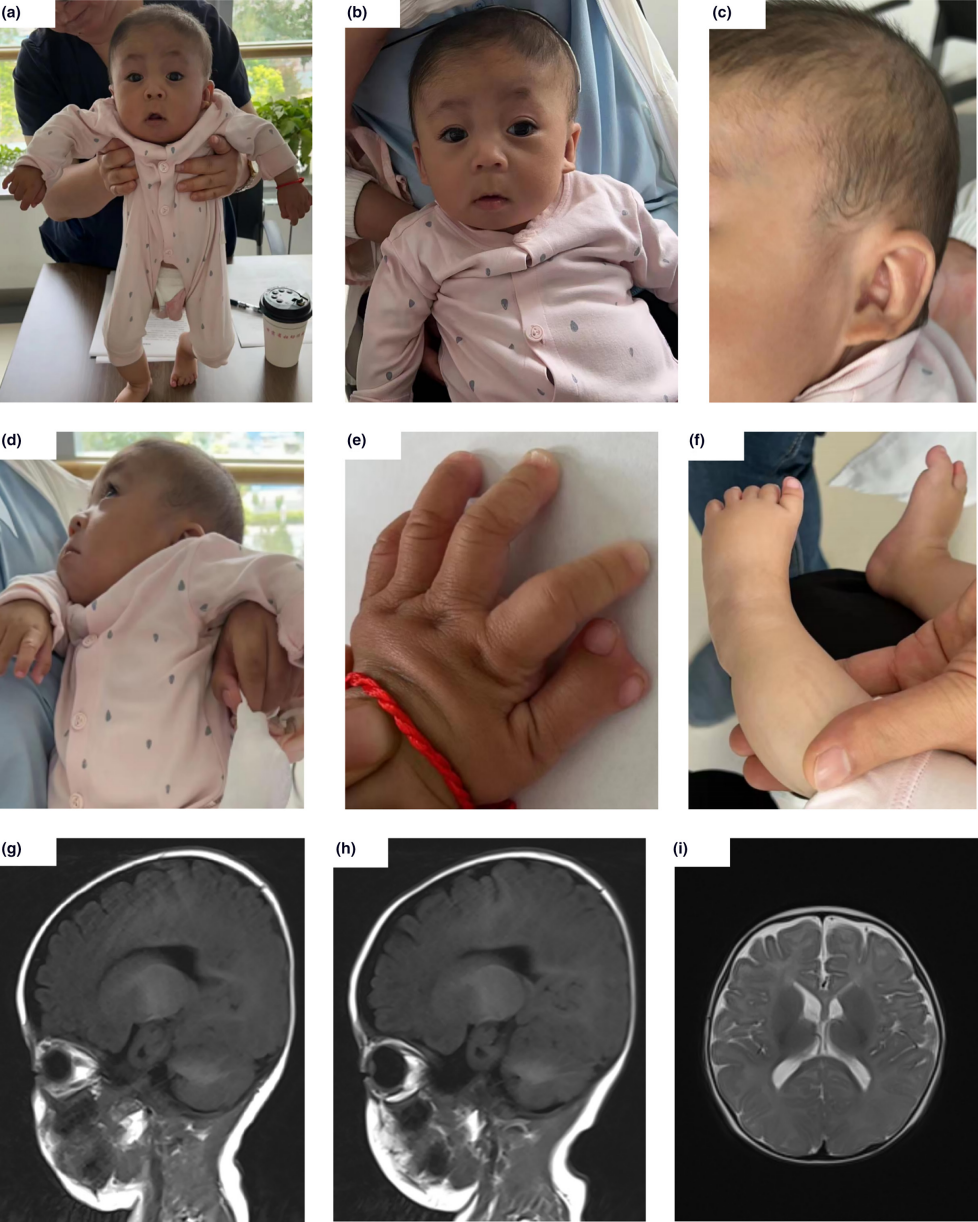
Clinical phenotypes of the patient. (a–f) Facial deformity: coarse face, micrognathia, high arched jaw, flat nose, long philtrum, and auricular deformity. (g–i) Features of cranial MRI (at the age of three months): hypoplasia of the corpus callosum.
